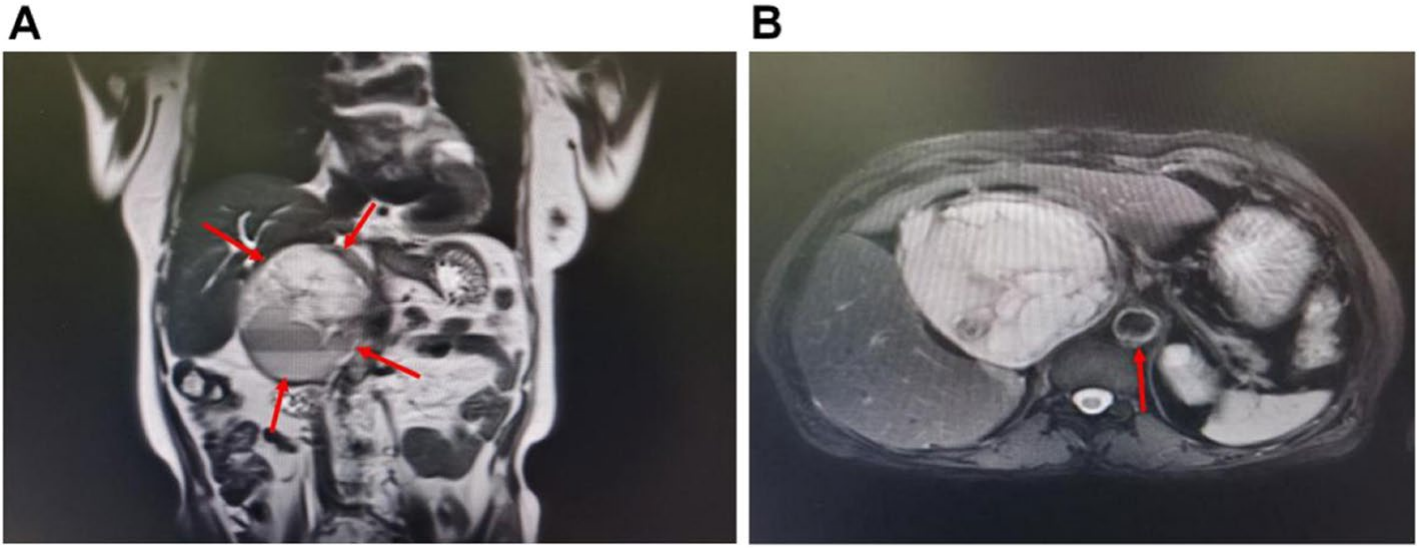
A, contrast-enhanced MRI scan showing a heterogenous mass of 11 cm in diameter in the right retroperitoneal region (arrows). B, contrast-enhanced MRI scan showing the giant mass pressing against the surrounding blood vessels; only the abdominal aorta is clearly visible (arrows); an axial view
